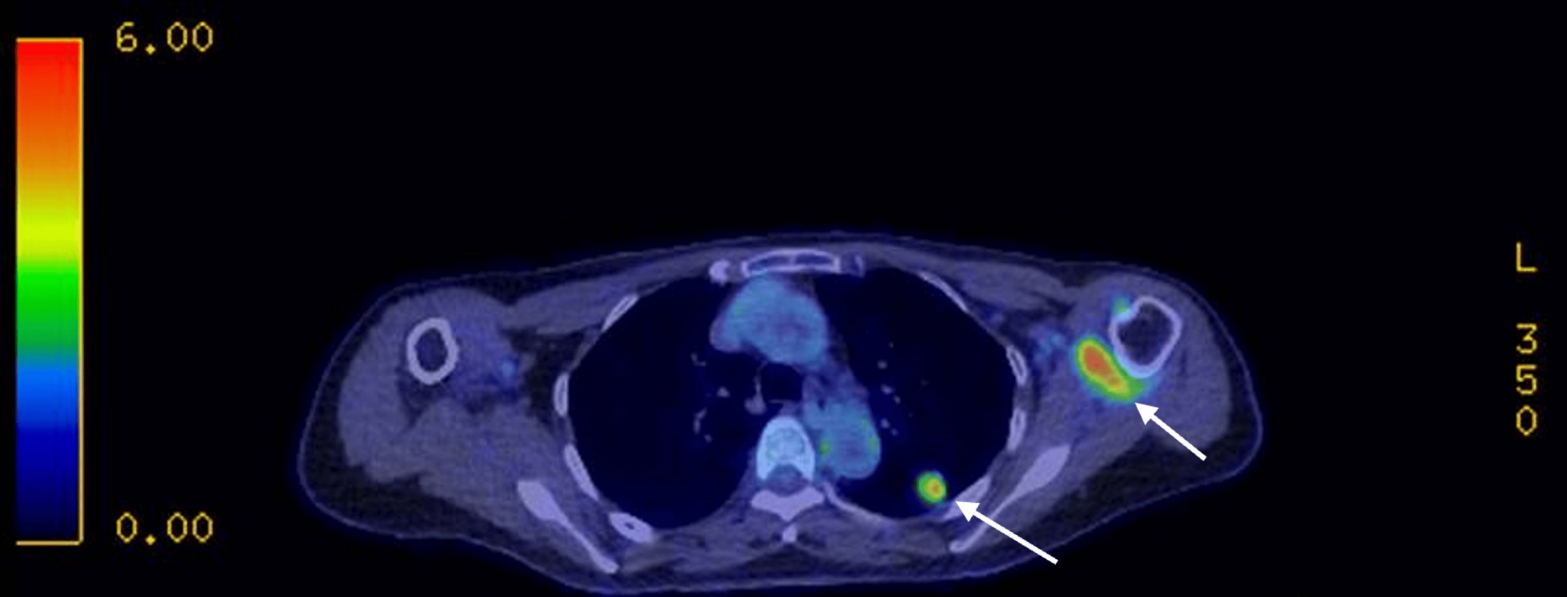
PET-CT showing the 16 mm FDG-avid nodule (SUV max 3.4) and two axillary lymph nodes showing low-grade uptake (SUV 3.0) Left arrow pointing to nodule and right arrow pointing to FDG-avid left axillary lymph nodes; FDG: fluorodeoxyglucose; PET-CT: positron emission tomography-computed tomography.
